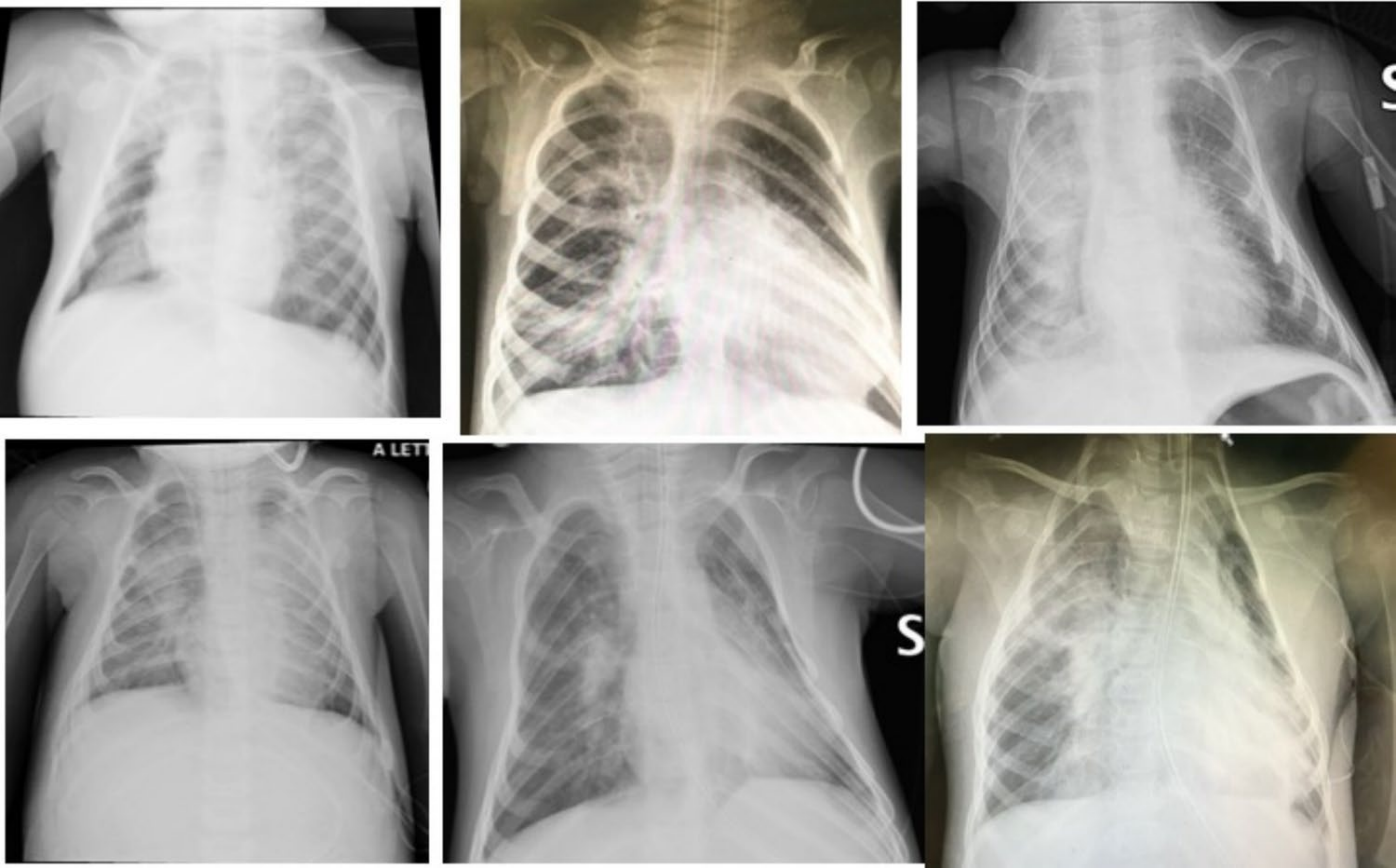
Chest X-rays appearance of type 1 SMA patients affected by ARDS
